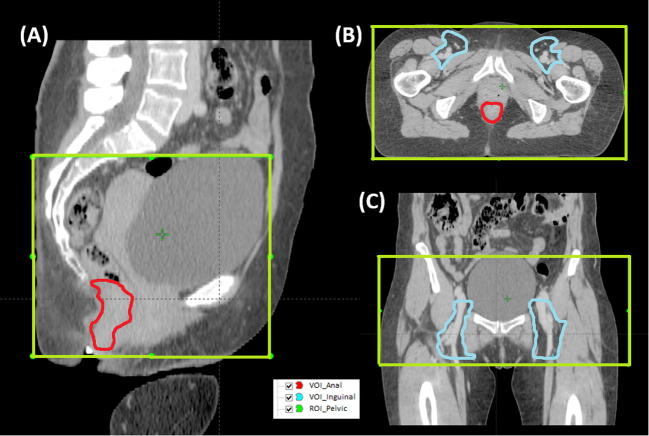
Planning CT scan demonstrating the VOI used for the primary tumour (VOI_Anal, red contour) and the inguinal nodes (VOI_Elective, light blue contour) and the ROI used for pelvic auto-match (green contour) in (A) Sagittal, (B) Axial and (C) Coronal slices.
